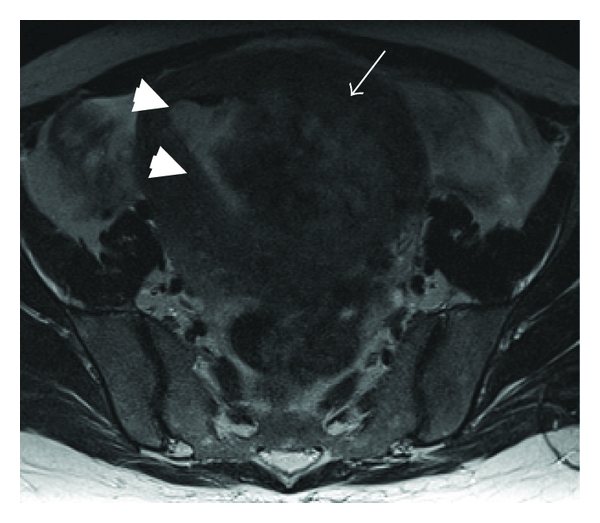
T2-weighted axial MRI image of the pelvis showing the large heterogeneous left intramural uterine mass (white arrow) encroaching into the uterine cavity (white arrowheads).
